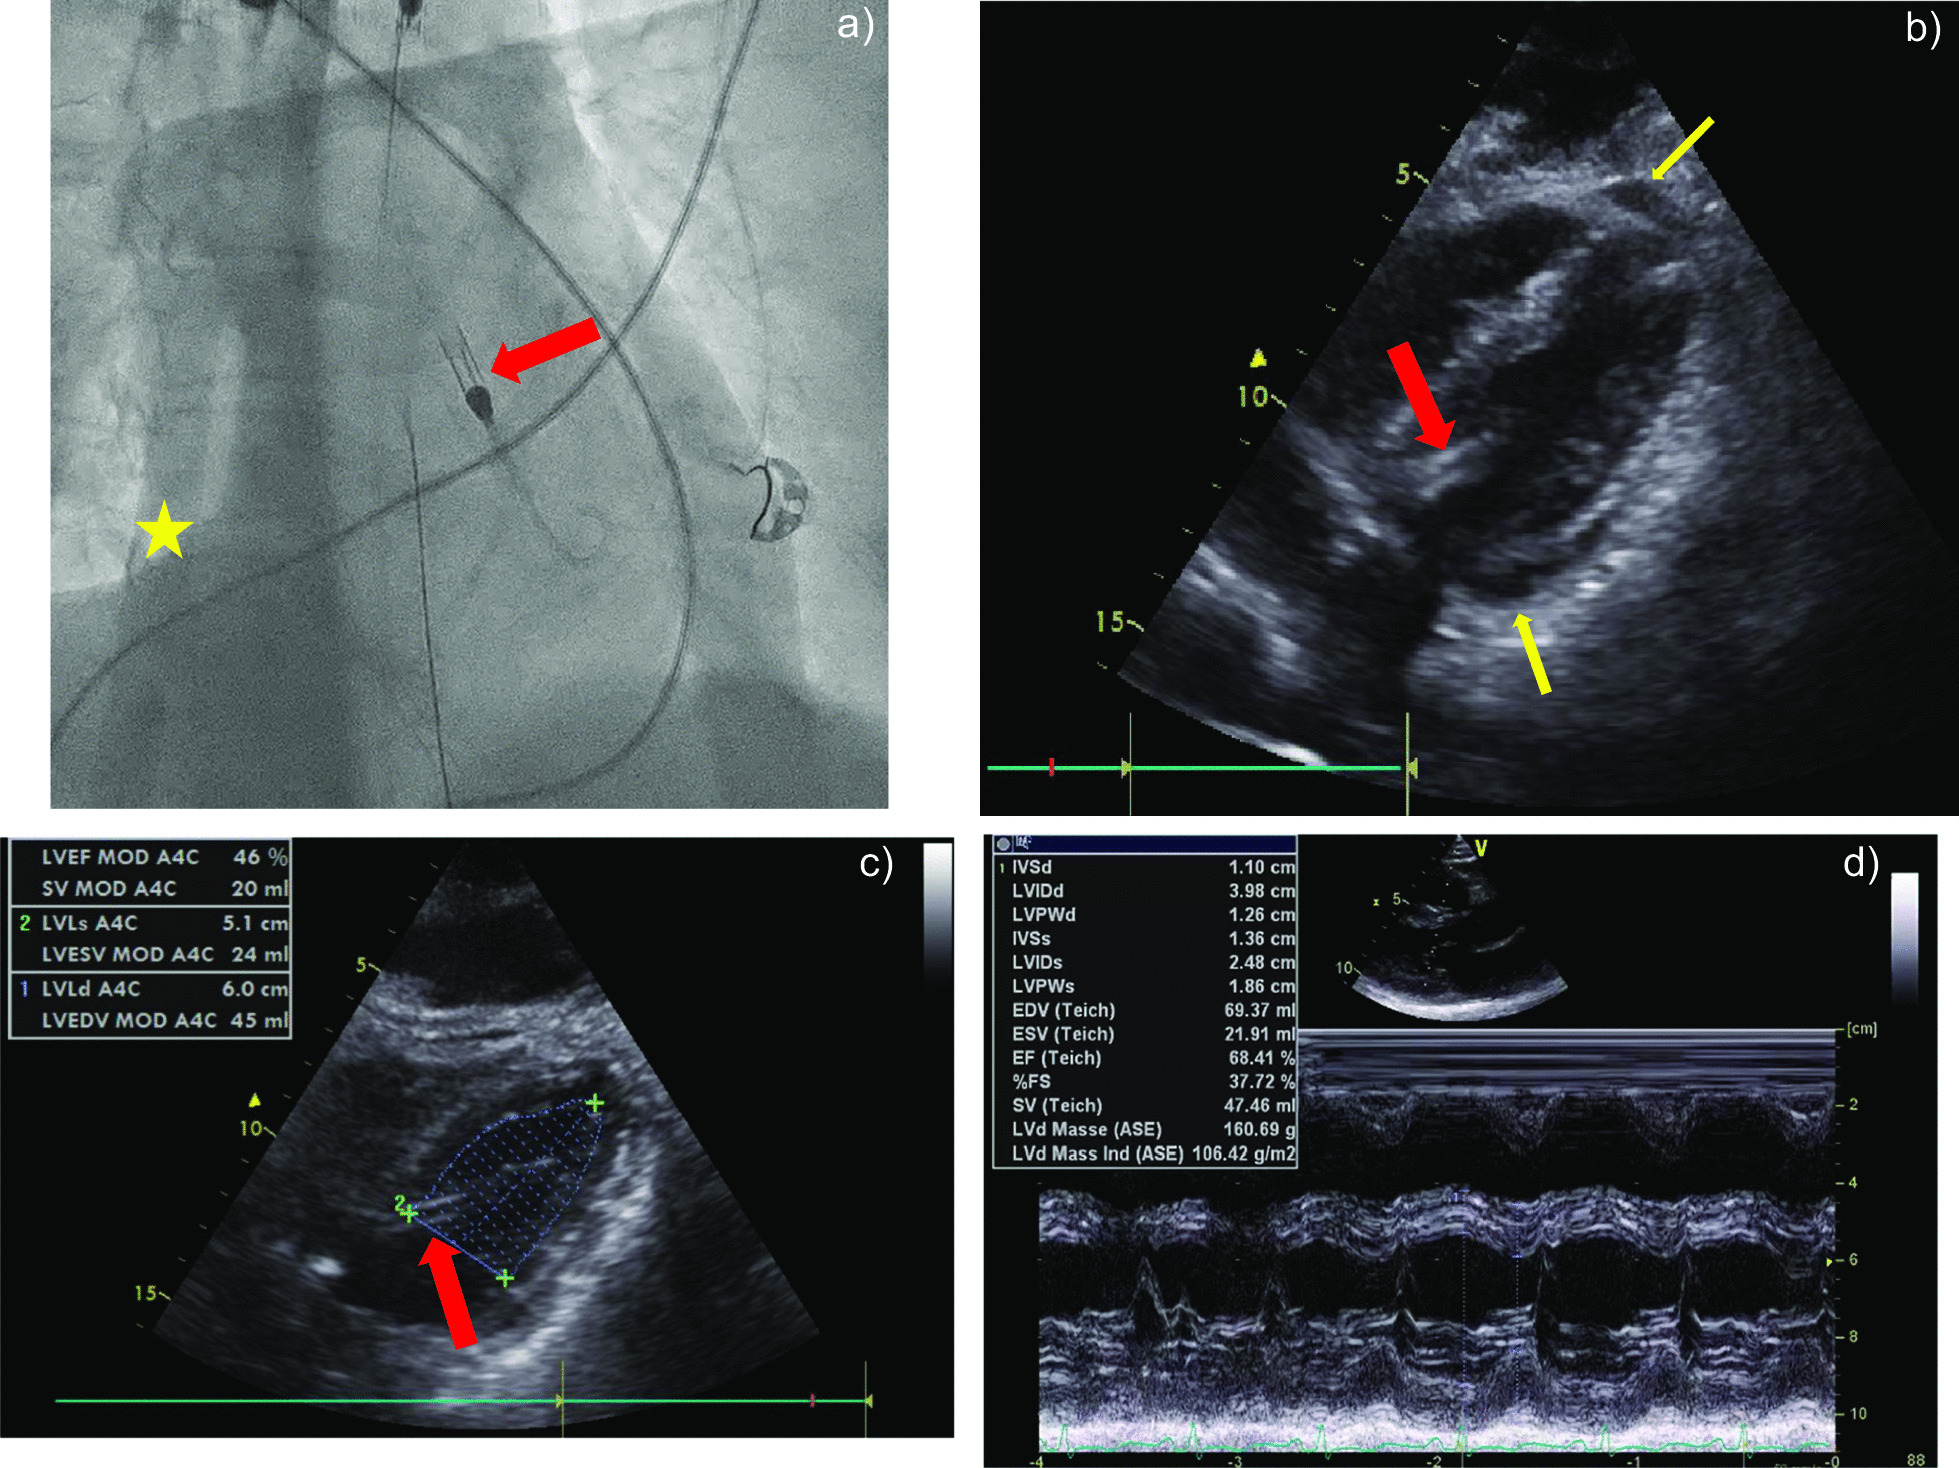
Aspects of ECMELLA treatment. a Fluoroscopy of the Impella CP implanted in the left ventricle (red arrow), and the venous cannula of the ECMO implanted in the inferior vena cava and in the right atrium (yellow asterisk). b TTE showing the severely hypokinetic left ventricle with the implanted Impella CP (red arrow) immediately after completion of the ECMELLA concept. Please note the non-compressing circular pericardial effusion (PE; yellow arrows). c TTE showing the risen LVEF (ca. 40%) 2 days after the initiation of ECMELLA. Please note the Impella CP in the LV (red arrow). d 2D echocardiography showing recovery of LVEF (68%) and LV dimensions after severe cardiogenic shock treated with ECMELLA. No residual PE is discernible
